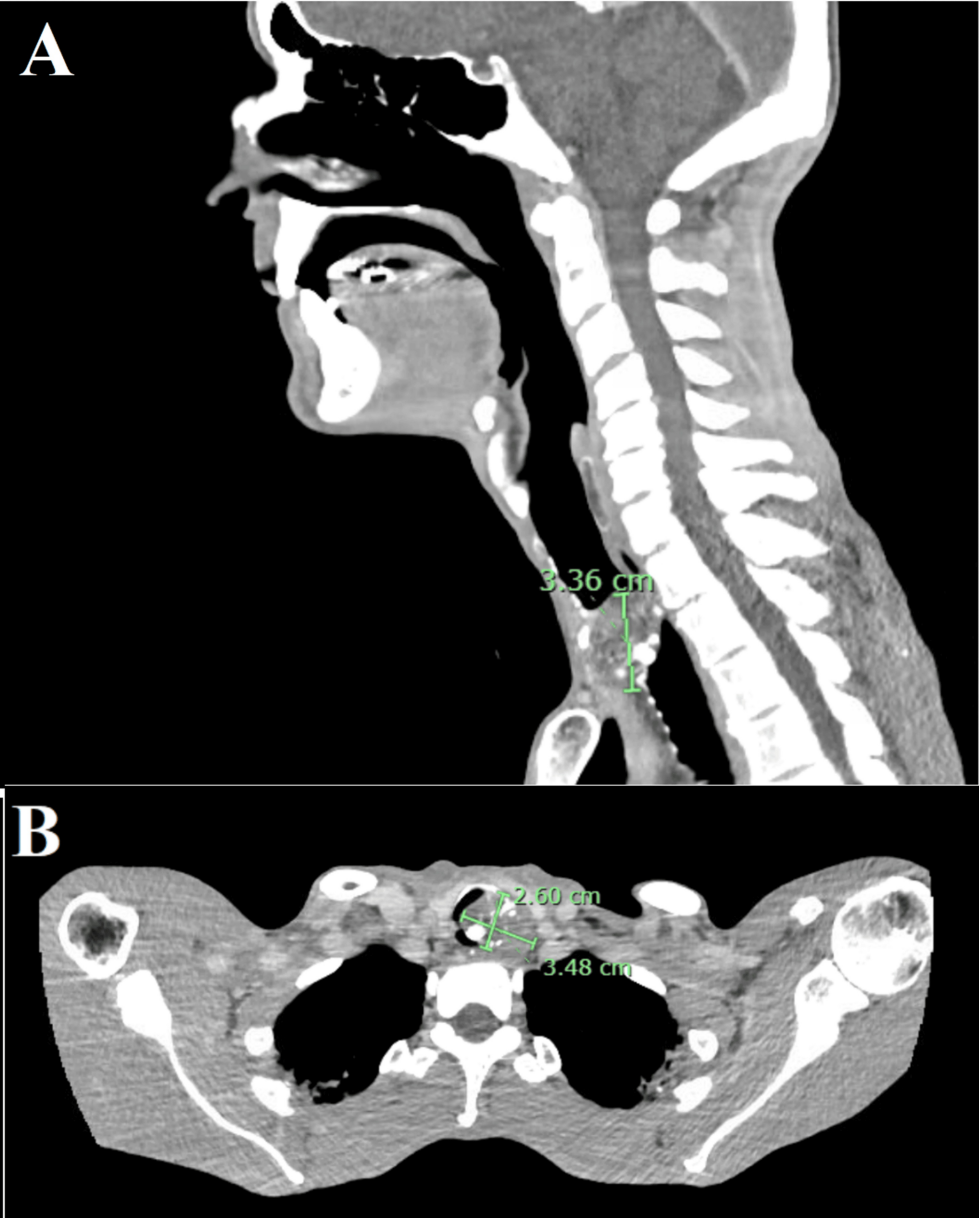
(A) Sagittal and (B) transverse contrast CT of the neck
